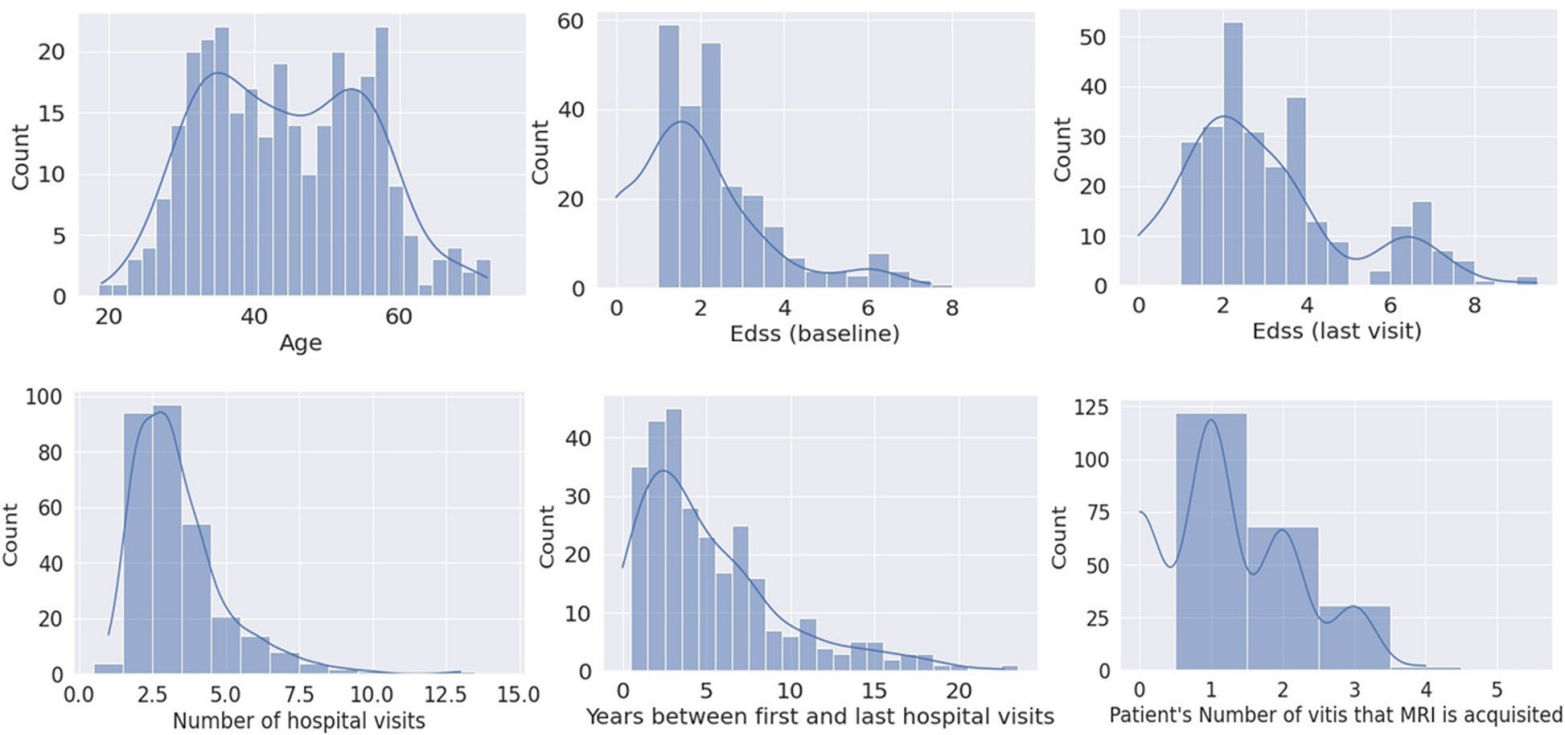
The histograms of all patients by age; baseline EDSS (at initial hospital visit); EDSS at the last hospital visit; total hospital visits; years between the first and the last hospital visit; number of hospital visits during which brain MRI scan was performed
